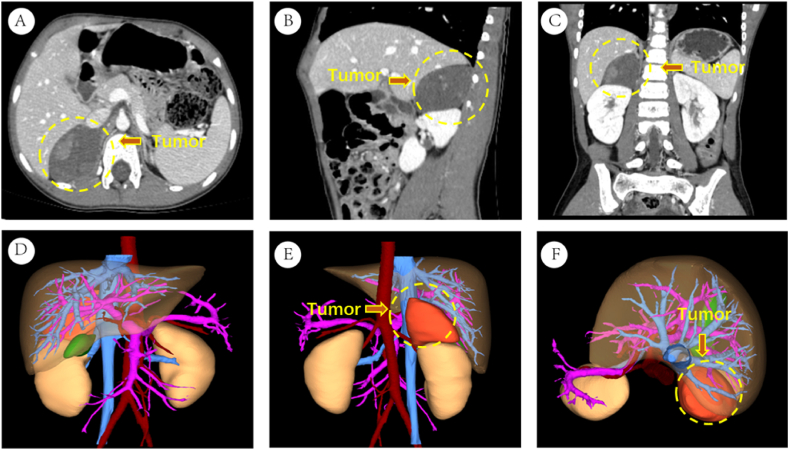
Presents the imaging data of proband 2. Figures A–C display abdominal CT images. Figures D–F show three-dimensional imaging pictures.
